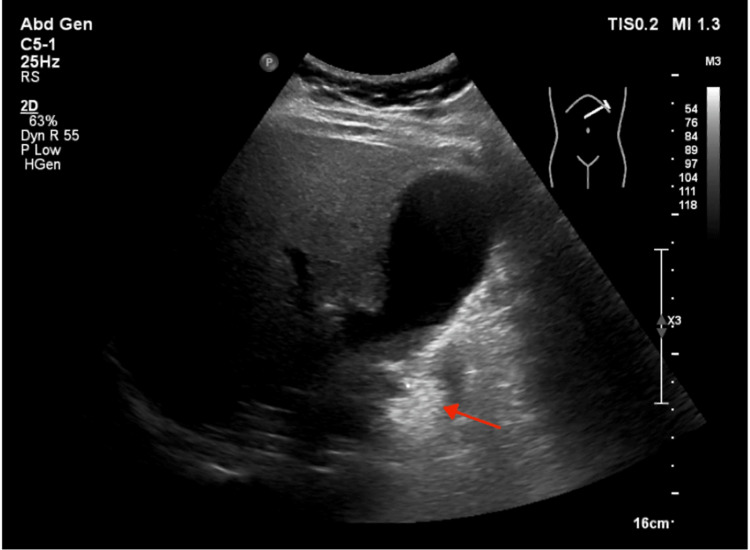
Axial grayscale ultrasound image with the arrow pointing to the small area of fat sparing
